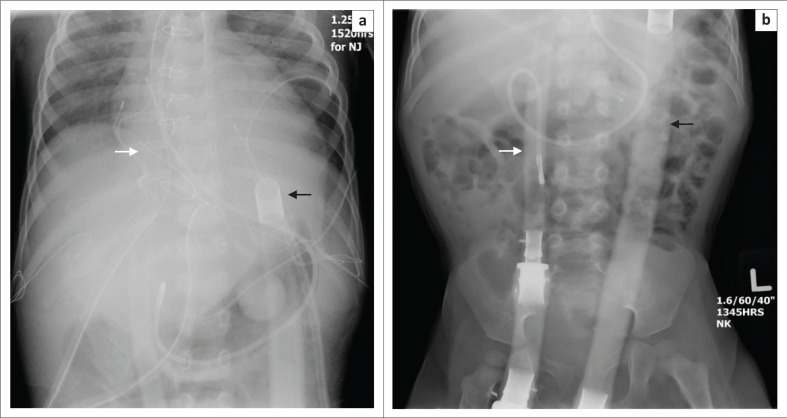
Chest (a) and abdominal (b) radiographs showing a Berlin Heart left ventricular assist device with its inflow (black arrow) and outflow (white arrow) cannulae implanted in the left ventricle and aorta, respectively, in a 2.5-year-old male child with severe left ventricular dysfunction following an episode of myocarditis.
